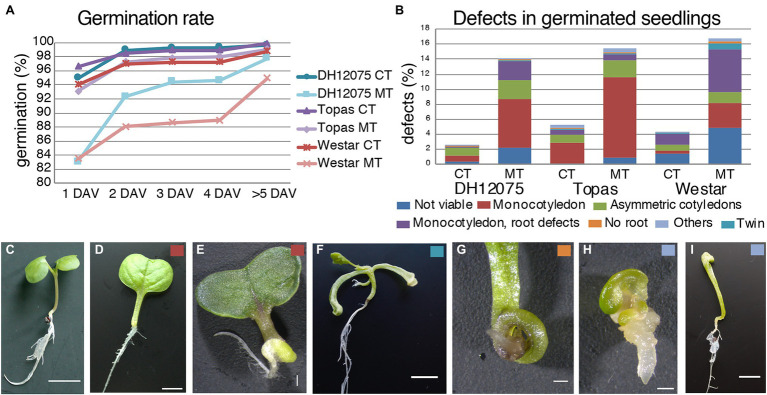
Seed development at high temperatures affects seedling viability. (A) Graph displaying the cumulative percentage of seed germination at 1, 2, 3, and 4 day after vernalization (DAV), and later for seeds produced by DH12075, Topas, Westar plants grown at CT and MT. (B) Percentage of defective seedlings per categories of defects: not viable (dark blue), with one cotyledon (red), asymmetrically positioned cotyledons (green), twin seedlings (turquoise), with one cotyledon, and defective root (purple), without any root (orange) and other categories (light blue). The analysis was performed on seedlings from seeds produced by DH12075, Topas, Westar plants grown at CT and MT. (C–I) Range of observed seedling phenotypes. A wild-type seedling is shown (C). Seedlings (D,E) are with one cotyledon (red). (F) The seedling is with two roots and two shoots (turquoise). (G) Zoom in of a seedling without root (orange). (H,I) Are shown seedlings from the other categories (light blue). The small colored square in the upper right corner refers to the categories. Scale bars represent 10 mm.
